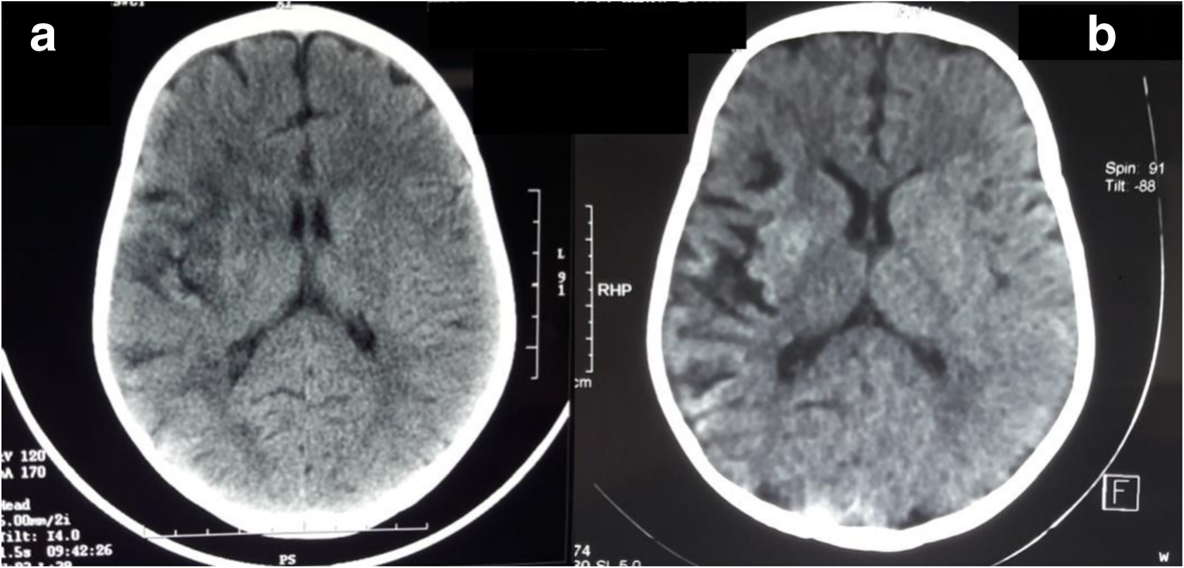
Brain computed tomography showing, at day 3 of deficit, a low density area in the right cortical and subcortical frontotemporoparietal regions and right insular cortex representing ischemic infarcts in the territory of the right middle cerebral artery (a). Evolution of previous ischemic lesion at day 9 of deficit (b)
